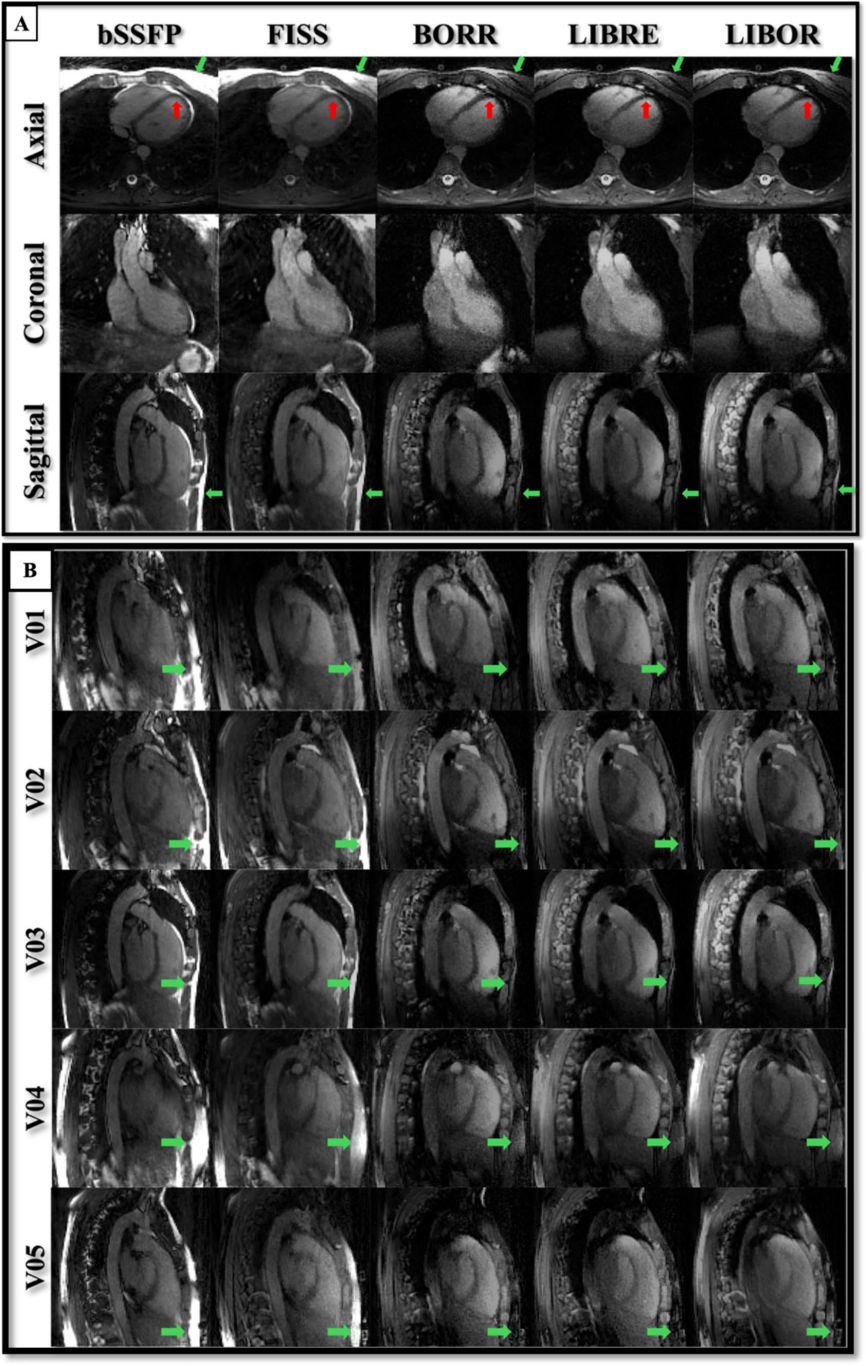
Cardiac and respiratory motion‐resolved 5D whole‐heart MRI reconstructed for volunteer data acquired with different fat suppression methods. (A) Representative images from one volunteer showing conventional non-fat-suppressed bSSFP, FISS, BORR-bSSFP, LIBRE-bSSFP, and LIBOR-bSSFP acquisitions. The images display the heart in the end-diastolic phase of the cardiac cycle and the end-expiration phase of the respiratory cycle. Red arrows show the myocardium wall, and green arrows represent subcutaneous fat. (B) Comparison of LIBOR-bSSFP acquisitions across all five volunteers (V01–V05), demonstrating consistent fat suppression performance across different subjects. Note that the perceived CNR is slightly reduced compared to previous studies due to the nominal RF excitation angle being limited to 50° in all volunteer experiments. The contrast window and level settings are consistent within each panel
